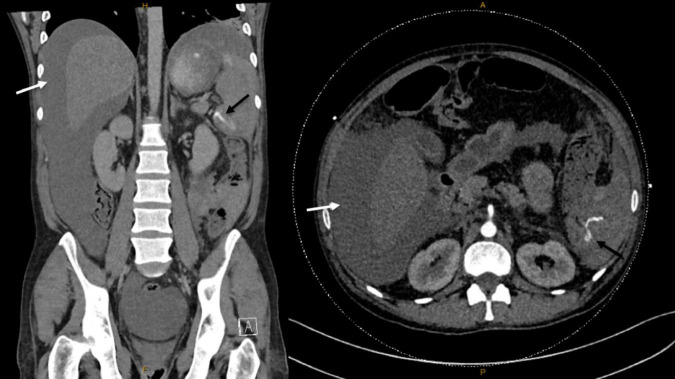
CT angiography (delayed venous and arterial phase) of the abdomen and pelvis. White arrow: significant intraperitoneal fluid accumulation due to hemoperitoneum. Black arrow: hyperdense foci due to acute bleeding from the inferior pole of the spleen with a surrounding subscapular splenic hematoma consistent with splenic rupture.
